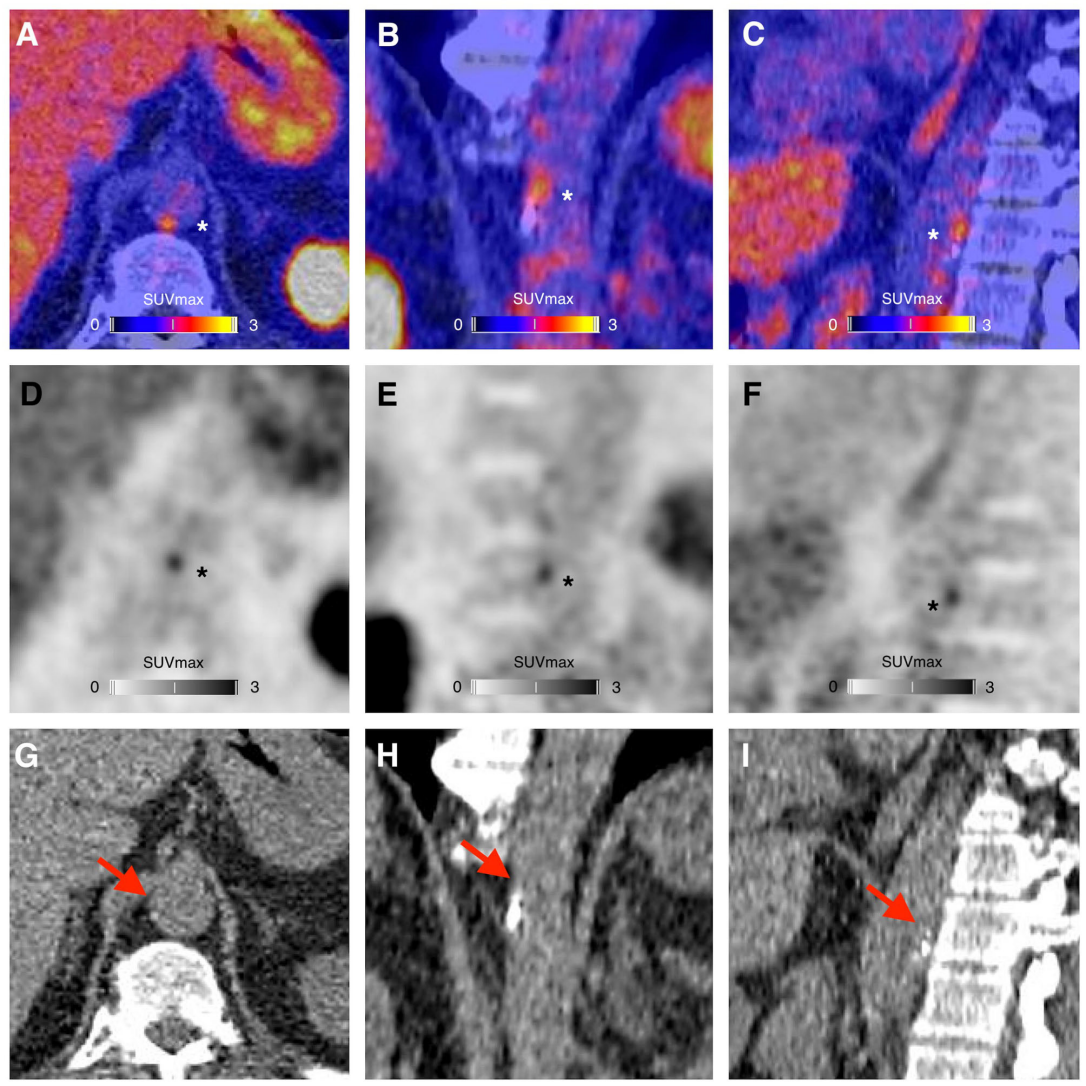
PET/CT (A–C), PET (D–F), and CT (G–I) images showing a focus of ^68^Ga-NODAGA-RGD arterial uptake (asterisks), in the periphery of a calcified atherosclerotic lesion (red arrows), in the wall of the abdominal aorta of a 63-year-old man who had a myocardial infarction with ST elevation 3 months before PET imaging. The risk factors of the patient were resumed to current tobacco use and family history of early coronary artery disease. At the time of the PET imaging the patient was treated with high intensity statin therapy, aspirin, ticagrelor, beta-blockers and an angiotensin-converting enzyme inhibitor, and was advised to adopt a healthy diet and to engage in regular physical activity.
